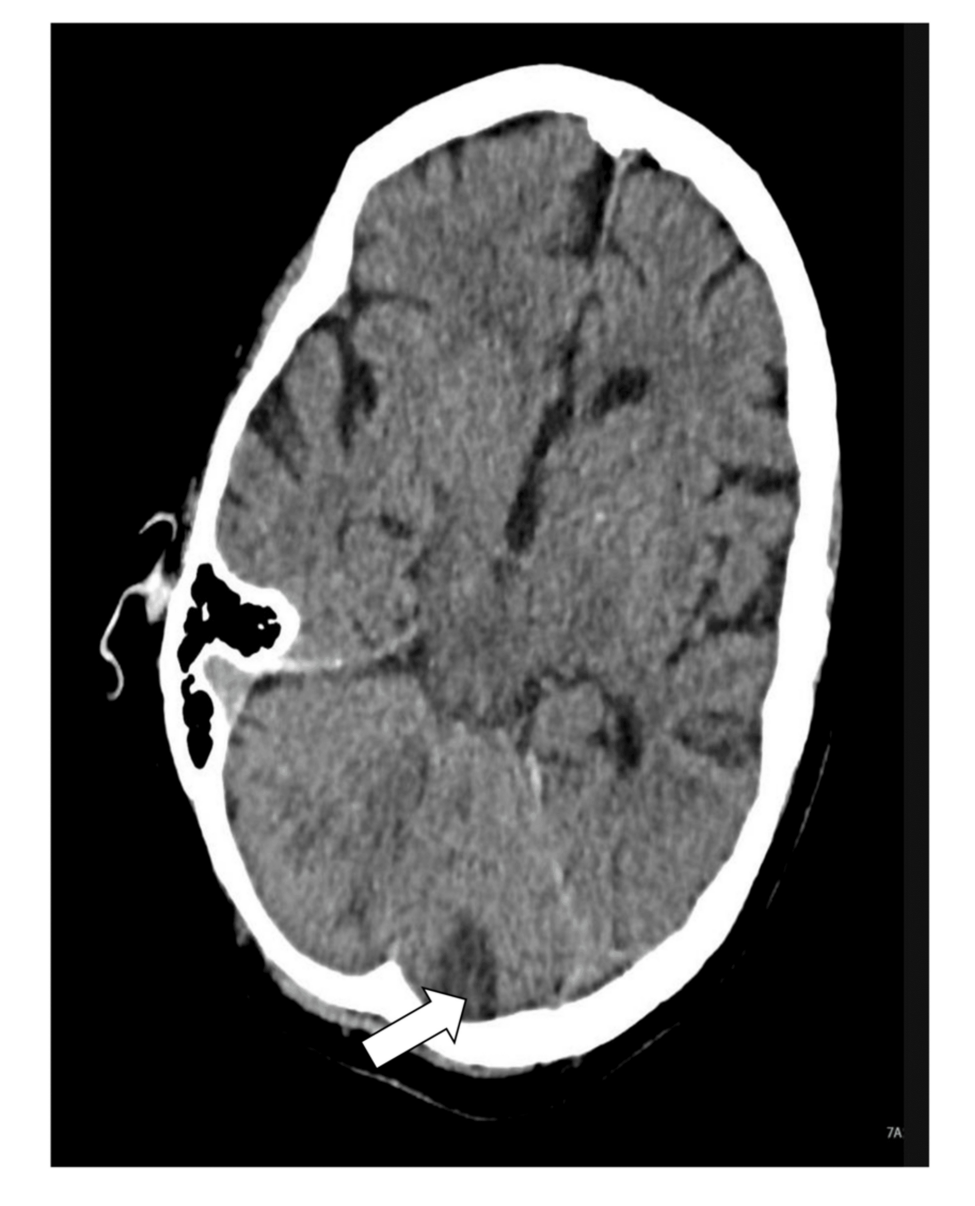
Axial CT brain demonstrating an acute infarct in the posterior aspect of the left cerebellar hemisphere, consistent with a posterior circulation stroke (white arrow)
